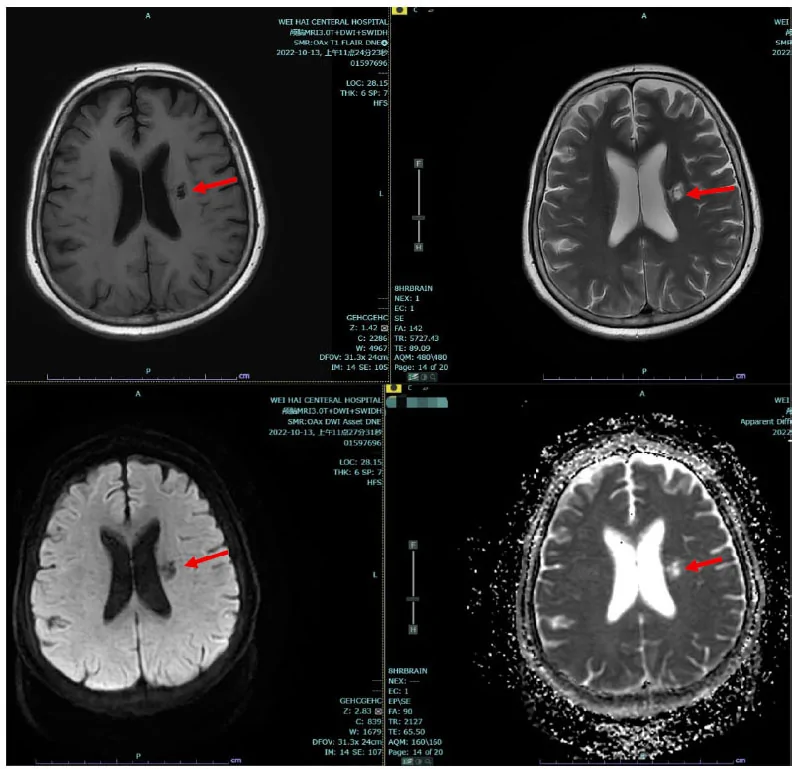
Brain MRI from October 13, 2022, shows patchy areas of prolonged T1 and T2 signal intensities in the left basal ganglia, with low signal on DWI and high signal on FLAIR.
